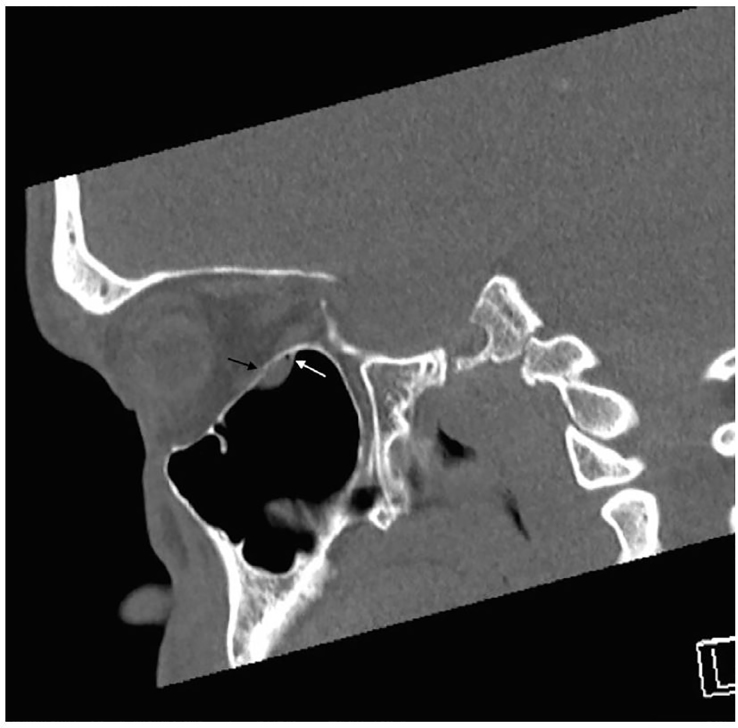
Sagittal non-enhanced CT in bone window: Subtle defect in orbital floor (black arrow) with small focus of air adjacent to soft tissue density (white arrow).
